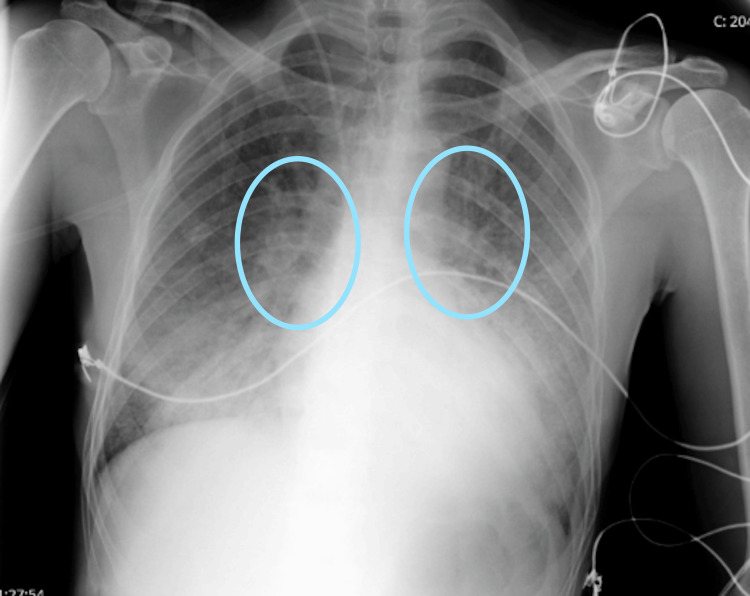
Chest X-ray on admission showing bilateral perihilar interstitial opacities, marked with blue ovals, consistent with diffuse pulmonary involvement in an immunocompromised patient.
